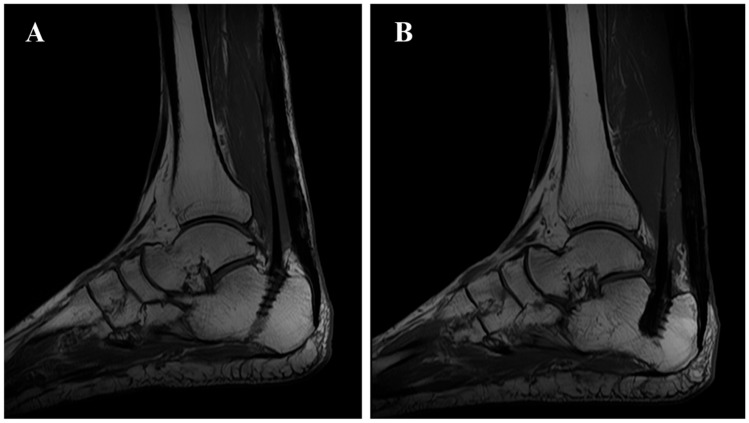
T1-weighted MRI at 12 months revealing full Achilles tendon continuity, with the transfer in place with tendon hypertrophy at the stump ((A) left foot; (B) right foot).
